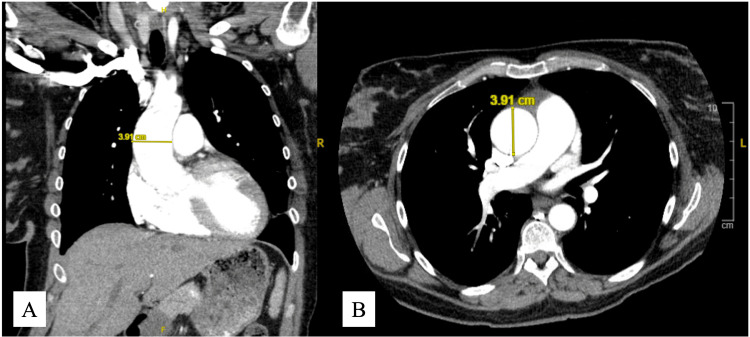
CECT of the thoracic aorta demonstrating mild dilation of the ascending aorta measuring 3.91 cm (A) Coronal view; (B) Axial view. The aortic contour is smooth without evidence of dissection, intramural hematoma, or penetrating ulcer. These images were obtained during evaluation of postpartum chest pain in a patient with known aortic valve insufficiency and newly reduced left ventricular systolic function. CECT: contrast-enhanced computed tomography
